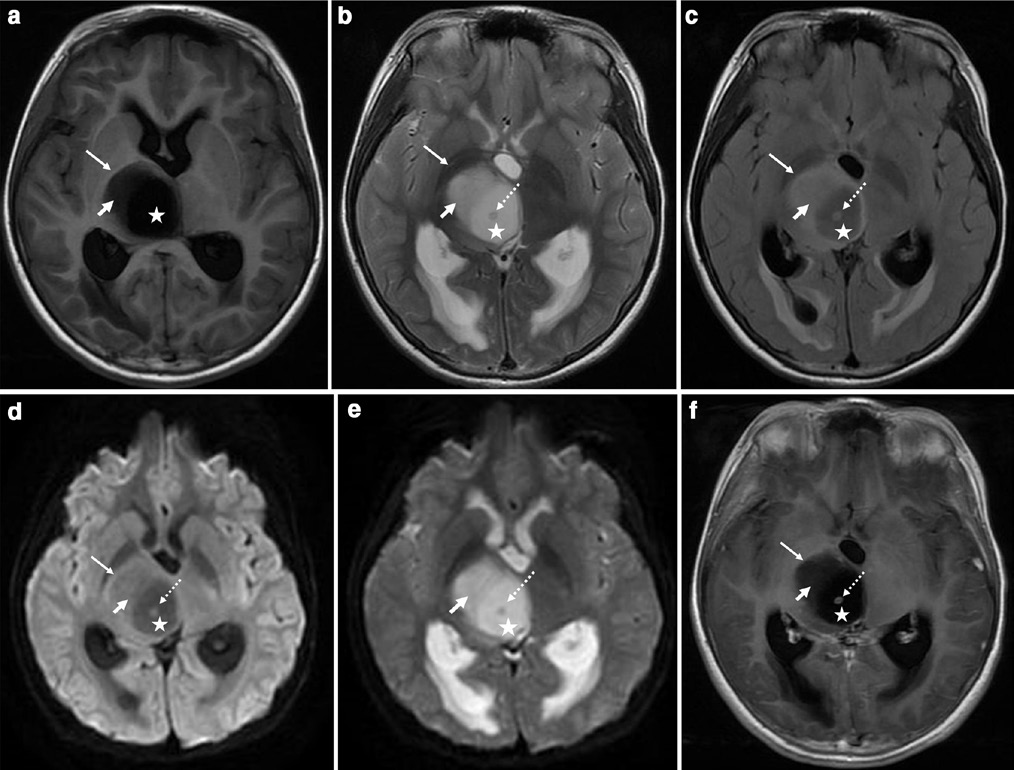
(A-F) Magnetic resonance images of the tumour (long white arrow). A and B, T1WI shows a well-defined solid cystic mass in the right thalamus. In the cystic part (pentagram), T1WI shows hypointensity, T2WI shows hyperintensity and slightly hyperintense nodules (white dotted arrow) are visible in the centre. The solid part (short white arrow) shows slight hypointensity in T1WI and slight hyperintensity in T2WI. C, Axial FLAIR shows slight hyperintensity in the cystic area and the central nodule, and the solid part shows hyperintensity. D and E, The lesion shows no diffusion restriction on diffusion-weighted imaging (DWI) and on the apparent diffusion coefficient map. F, Axial T1WI enhancement shows the annular enhancement of central nodule. (MRI = magnetic resonance imaging, T1WI = *T*_1_-weighted1-weighted images, and T2WI = *T*_2_-weighted2-weighted images).
